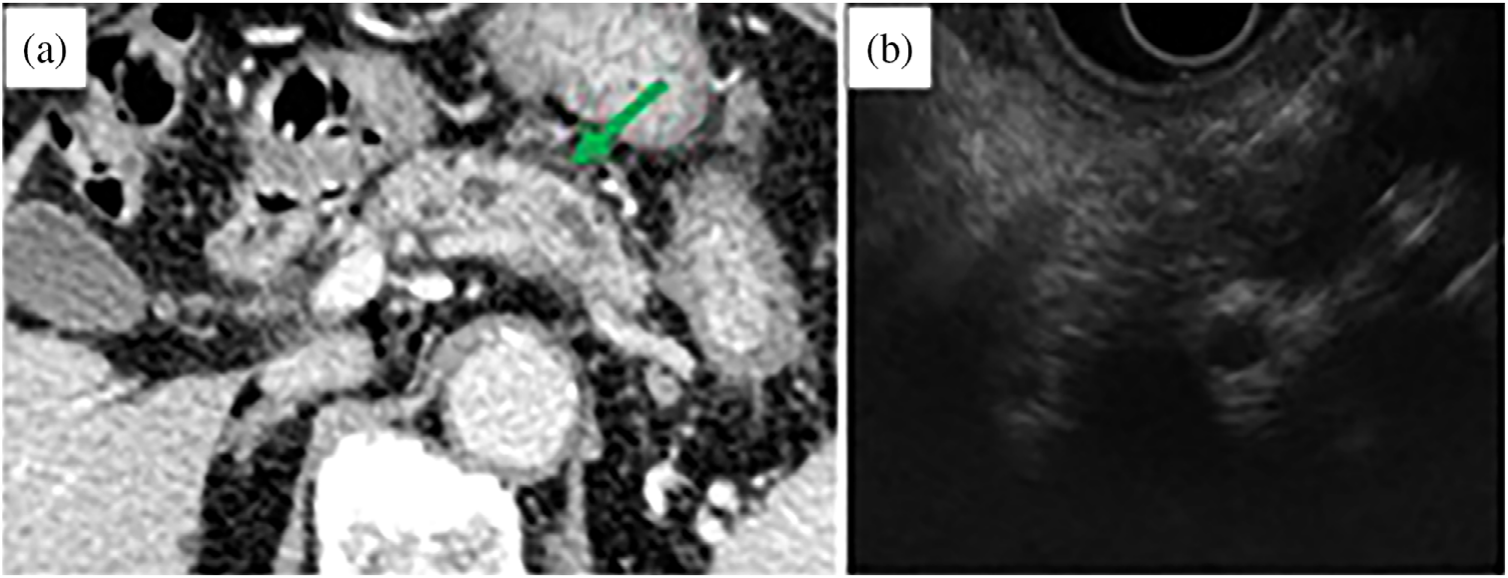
(a) The main pancreatic duct is disrupted in the pancreatic body, with a dilation of the distal main pancreatic duct. Contrast‐enhanced computed tomography shows a poor enhancement in the same region. (b) Endoscopic ultrasound shows an irregularly shaped hypoechoic mass, which is punctured.
